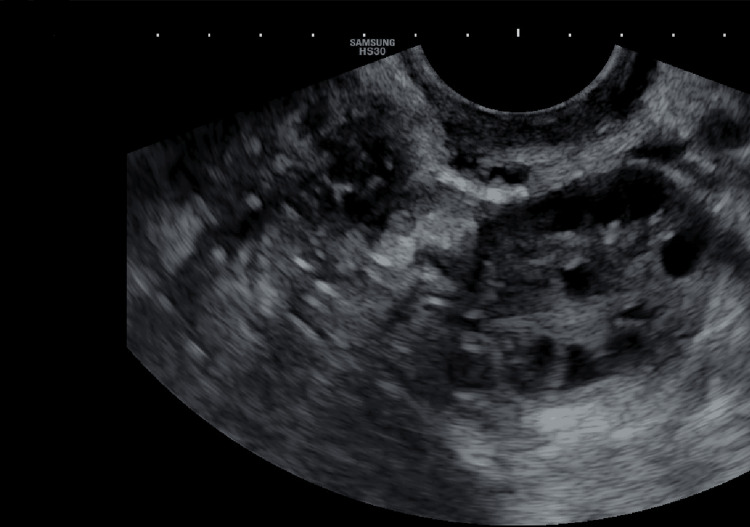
Transvaginal sonography image of the patient's left ovary on day two of the menstrual cycle
